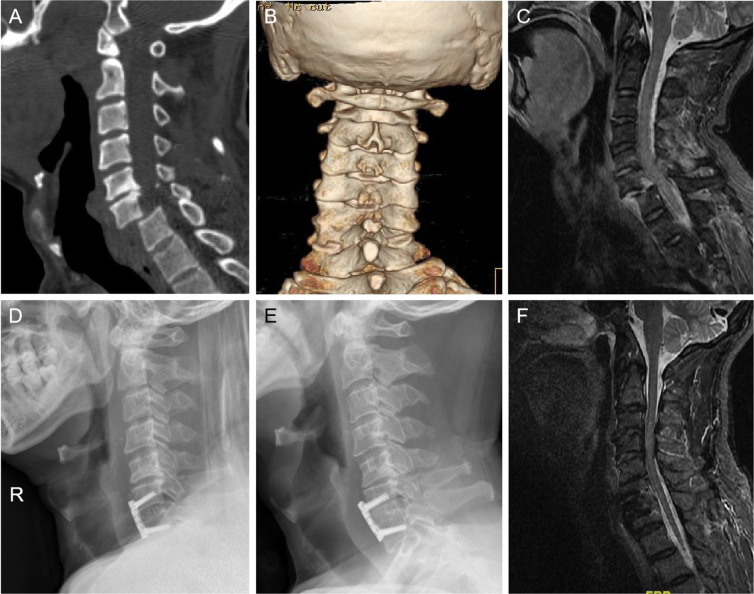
Imaging of anterior surgery. A 58-year-old man with C6/7 dislocation (A). 3D-CT showed the right facet was interlocked (B). X-Ray showed satisfactory reduction (D). No obvious loss of the lordosis angle in C6/7 level at 11 years after surgery (E). MRI showed no spinal stenosis in C6/7 level, and the spinal cord signal was normal (F).
